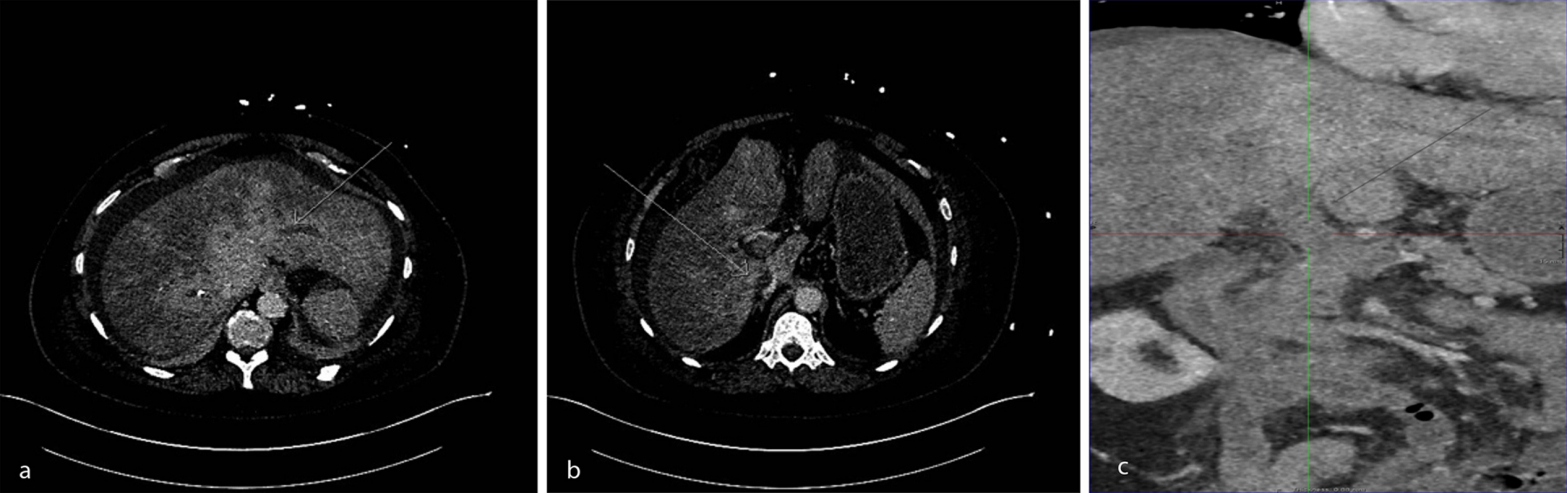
Pre-procedure contrast-enhanced CT scan of the abdomen in the portal venous phase demonstrating occlusive thrombus in the (a) hepatic veins (arrow), (b) inferior vena cava (arrow) and (c) main portal vein (arrow), extending into the splenic vein and superior mesenteric vein. All the images demonstrate a background hepatic parenchymal congestion with a mosaic enhancement pattern, giving a “nutmeg” appearance.
